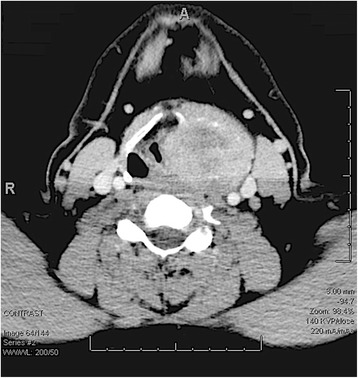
CT with contrast of the neck showing a large laryngeal mass destroying the left thyroid cartilage
